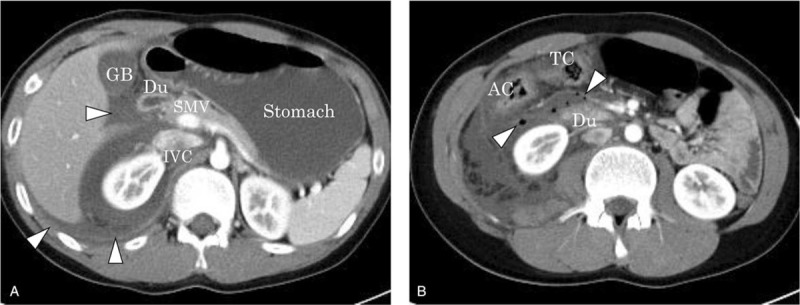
Pre-hospital computed tomography findings. (A) Image depicting fluid retention and dilatation of the stomach. Fluid retention around the hepatorenal recess space, also referred to as the pouch of Morison, was revealed (white arrowheads). Du = duodenum, GB = gallbladder, IVC = inferior vena cava, SMV = superior mesenteric vein. (B) Image depicting the accumulation of luminal gas within the posterior peritoneal cavity around the duodenum (white arrowheads). AC = ascending colon, TC = transverse colon.
